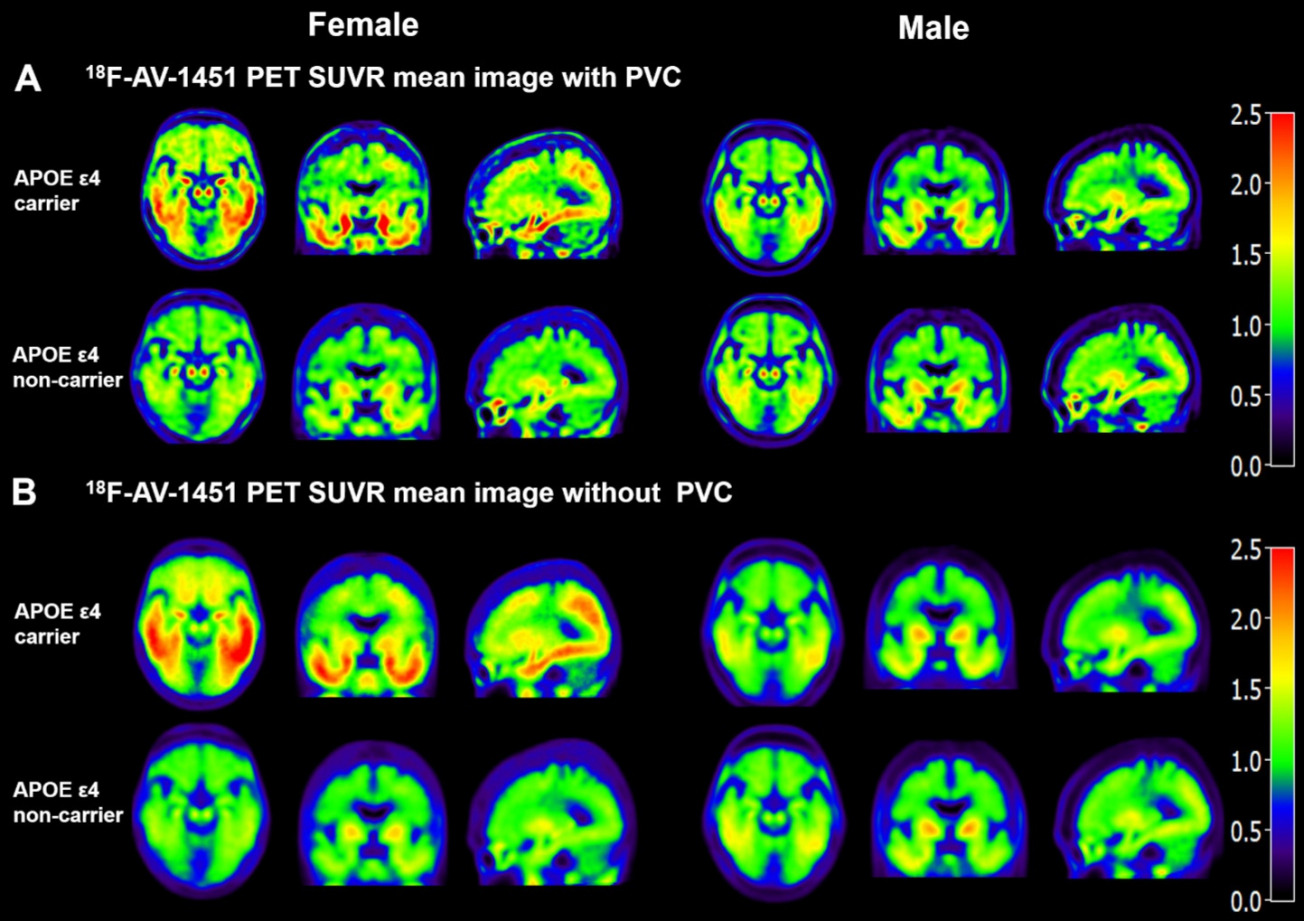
Mean SUVR images of^ 18^F-AV-1451 PET with and without partial volume correction in individuals with AD. Partial volume corrected SUVR images (A) show increased contrast and spatial resolution compared with the images without PVC (B). Both with and without PVC images visually demonstrate that FACs have higher ^18^F-AV-1451 SUVR. FACs: female APOE ε4 carriers, PVC: partial volume correction.
